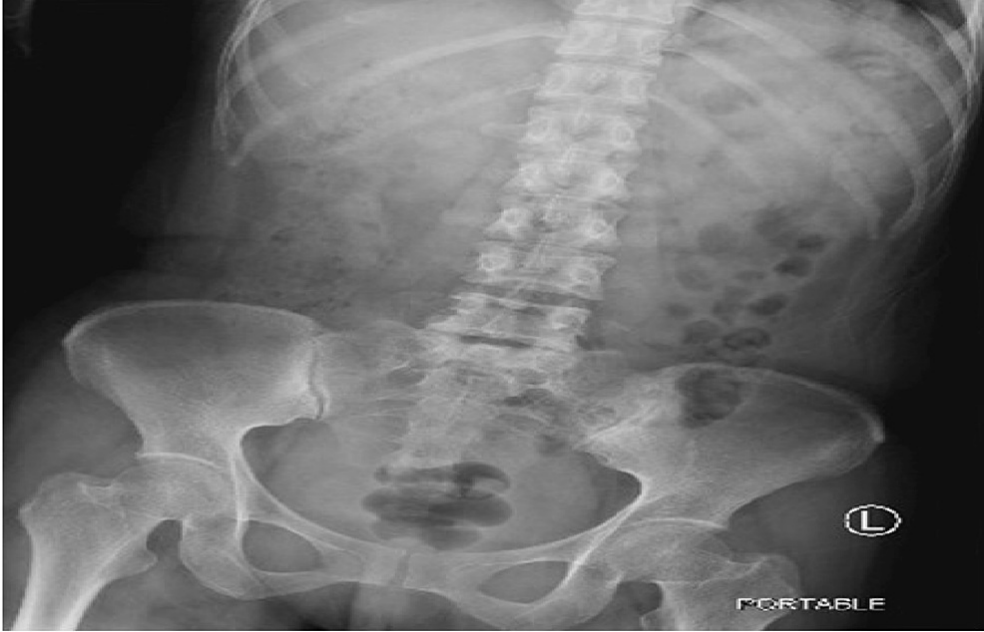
X-ray abdomen fails to identify any radiopaque substances.
